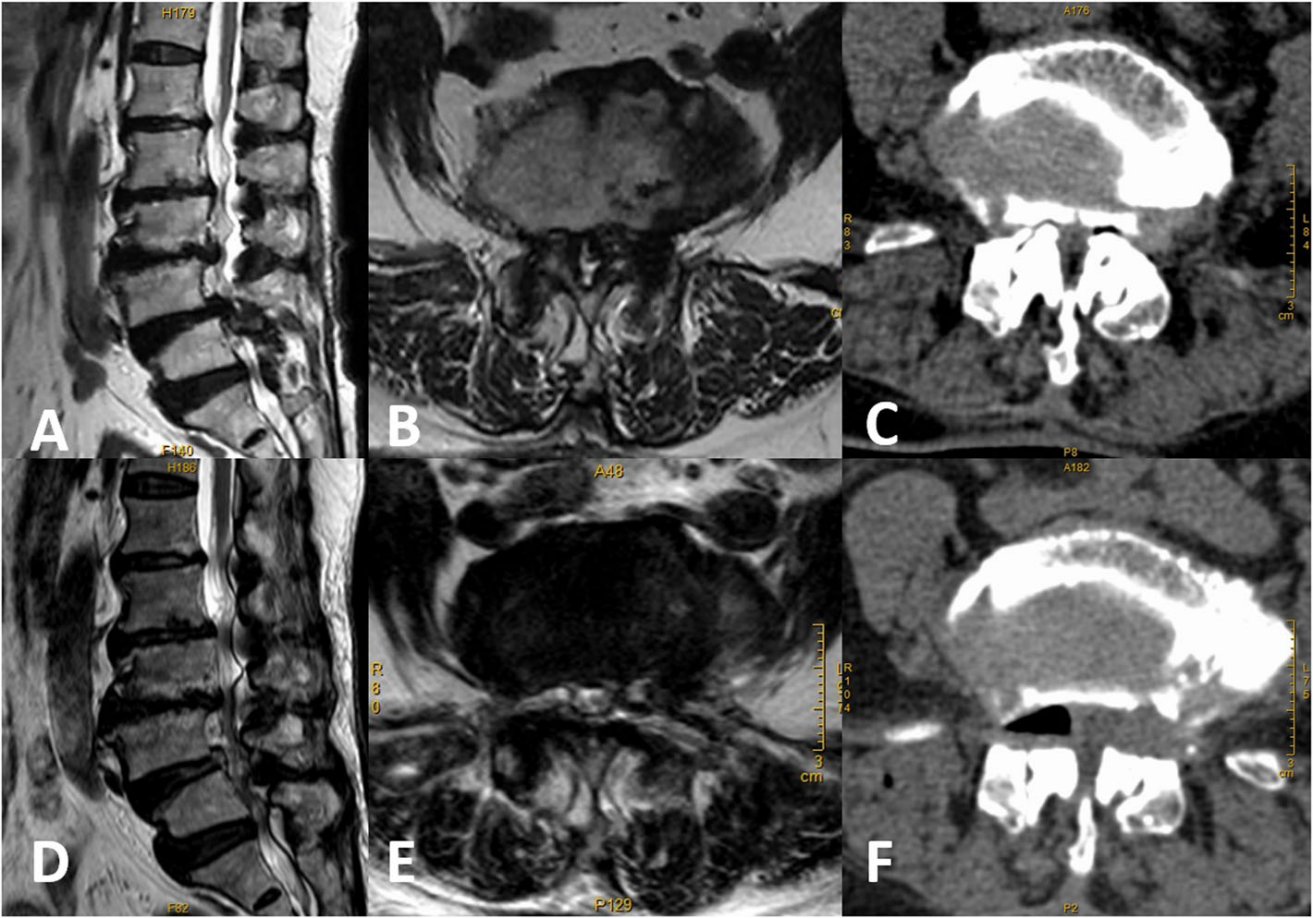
a-b Central spinal stenosis of L4–5 was shown in the preoperative MRI, the cross-sectional area of the dural sac was 27.16mm^2^; c Preoperative CT showing bilateral bony lateral recess stenosis; d-e Postoperative MRI showing that the central spinal canal was decompressed, the cross-sectional area of the dural sac was 98.86mm^2^; f Postoperative CT showing that the distal decompression reached the upper bony lateral recess (zone 2) and both the facet joints were well preserved
